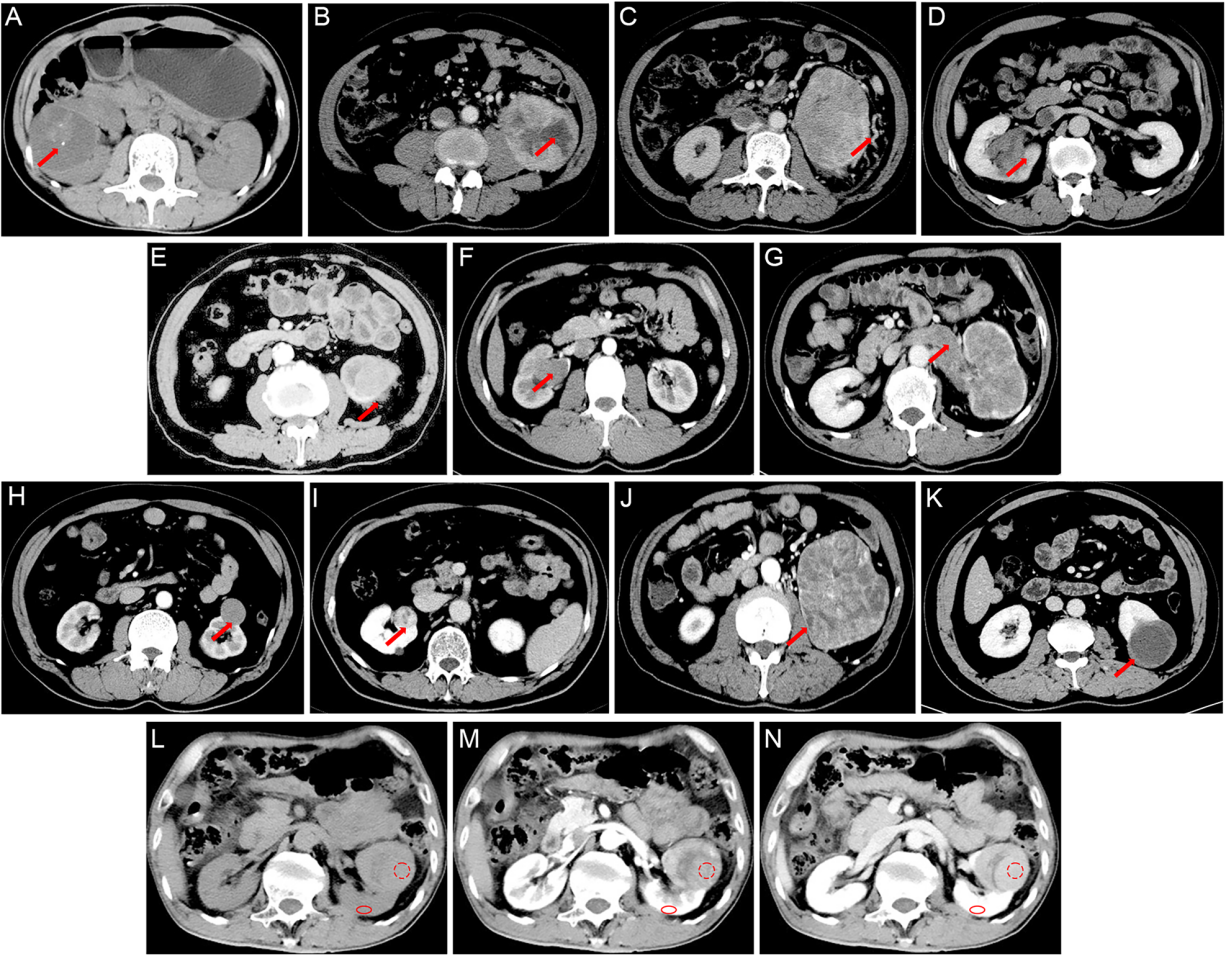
Display of image features. A Calcification, B CT necrosis, C peritumoral neovascularity, D CT renal sinus fat invasion, E CT perinephric fat invasion, F CT collecting system invasion, G CT vein invasion, H homogeneous enhancement, I solid or predominantly solid lesions with small areas of low attenuation, J lesions with mixed solid and low-attenuation areas, K predominantly low-attenuation lesions with peripheral enhancement. L–N The placement method of ROIs for measuring TEV and CEV. TEV, tumor enhancement value; CEV, cortex enhancement value
